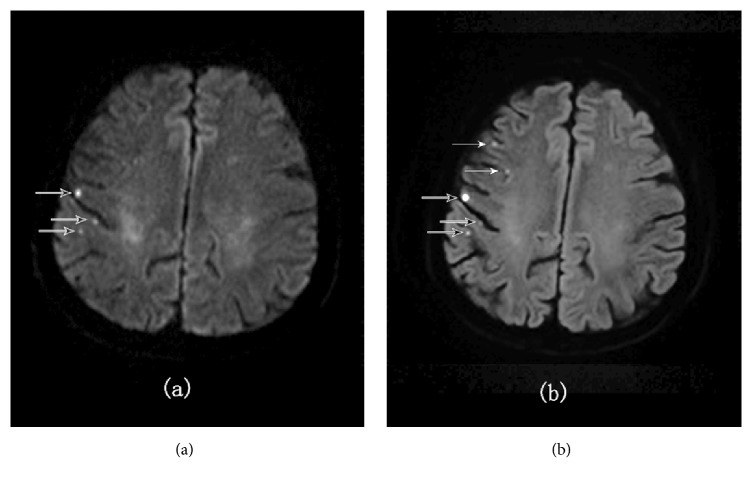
A 49-year-old male patient with acute cerebral infarction showed increased sensitivity of rs-DWI. (a) On ss-DWI image, 3 subtle lesions (black arrows) were found: 1 lesion was at the right frontal cortex, and 2 lesions were at the right parietal cortex. (b) On rs-DWI image, 2 more subtle lesions (white arrows) were found.
